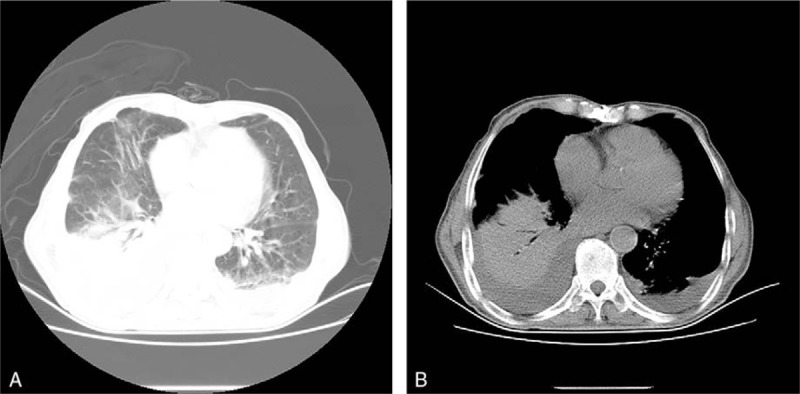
A, B, CT scan of the lung showing (A) bilateral compressive atelectasis with large pleural effusions in lung window and (B) bilateral large layering pleural effusions in mediastinal window.
